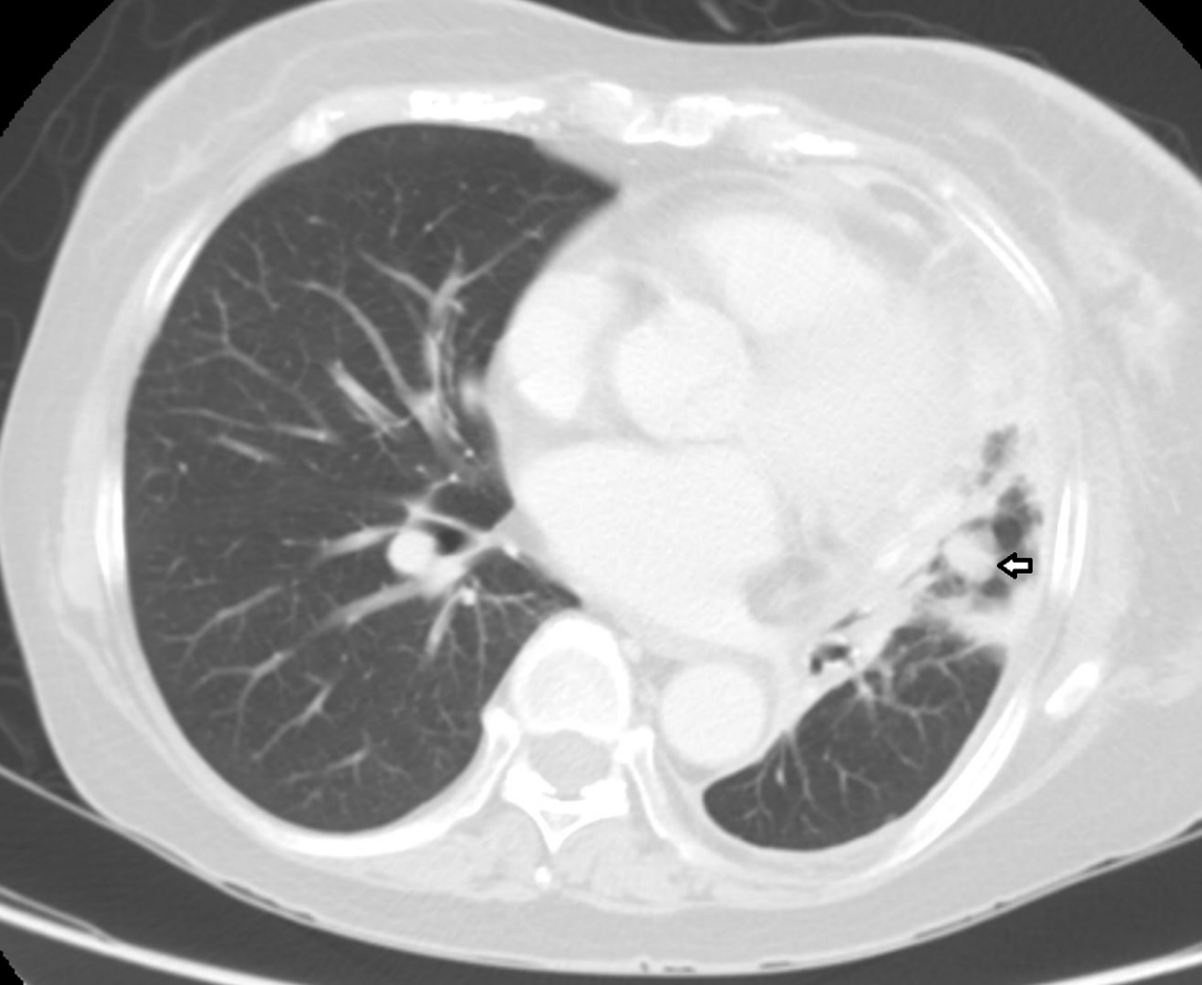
CT scan in the lung window showed a mass in the lingula lobe of the lung, in keeping with a primary tumour.
